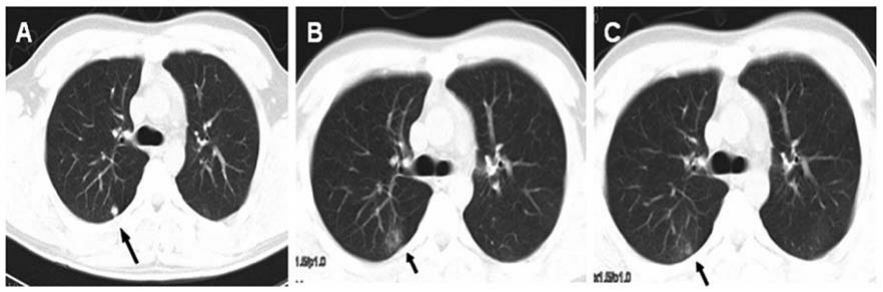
Radiation pneumonitis occurs 2 months after SRS treatment. Before SRS treatment (A). Two months after SRS treatment (B), CT scan showed radiation pneumonitis (black arrow) without any complaint from the patient. (C) Without medical intervention, radiation pneumonitis resolved two months later.
